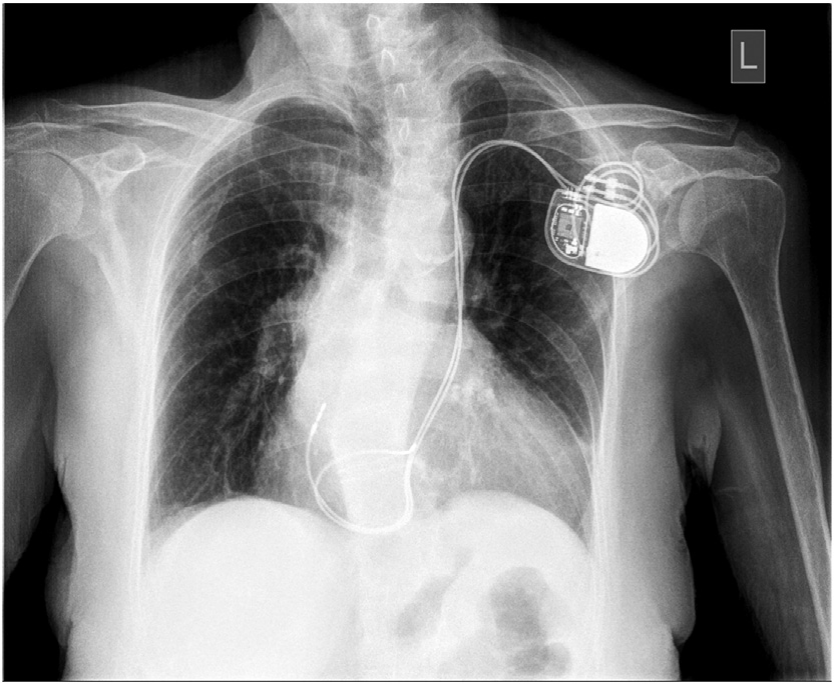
X-ray image of implanted cardiac pacemaker in patient with persistent left superior vena cava (anteroposterior view).
